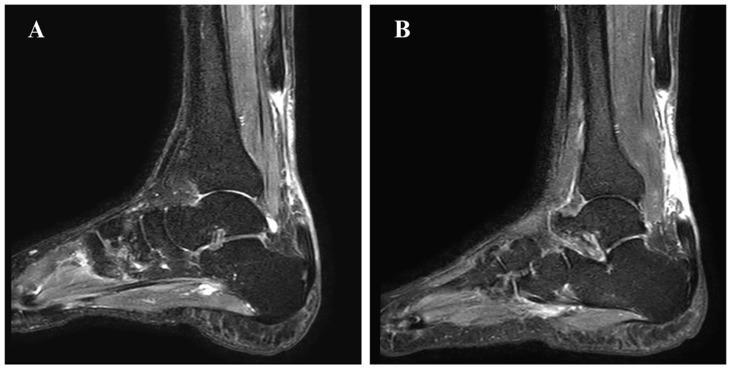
Pre-operative MRI (T2-weighted) revealing tendon rupture with significant retraction ((A) left foot 7.0 cm gap; (B) right foot 7.5 cm gap).
